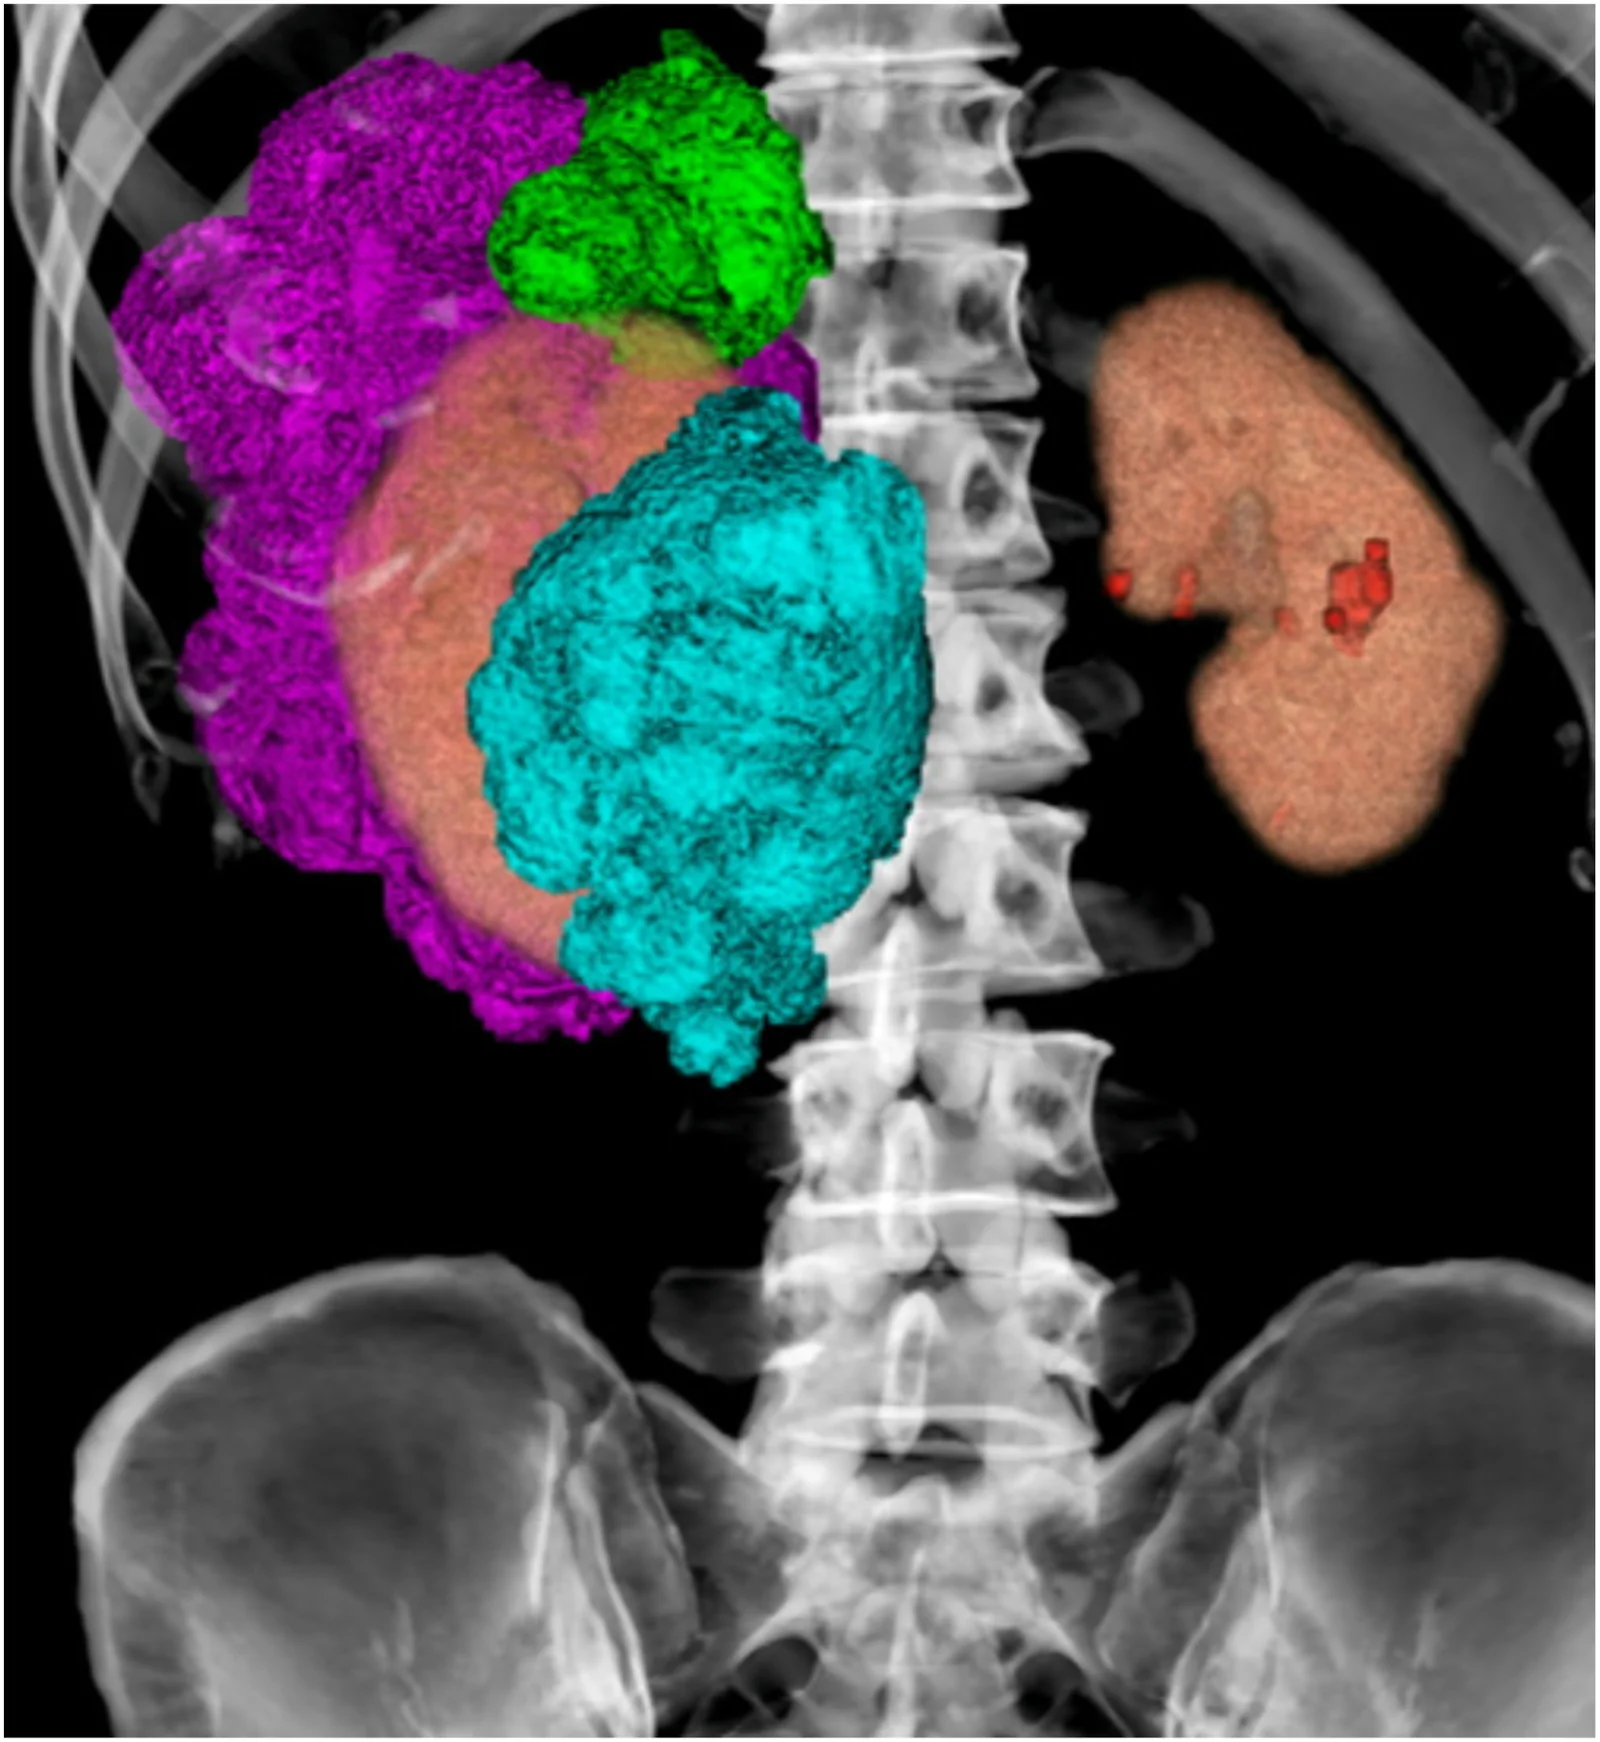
Volume-rendered CT image demonstrating bilateral multifocal renal angiomyolipomas. The three large right renal lesions are highlighted in pink, green, and light blue, whereas multiple small left renal lesions are shown in red.
